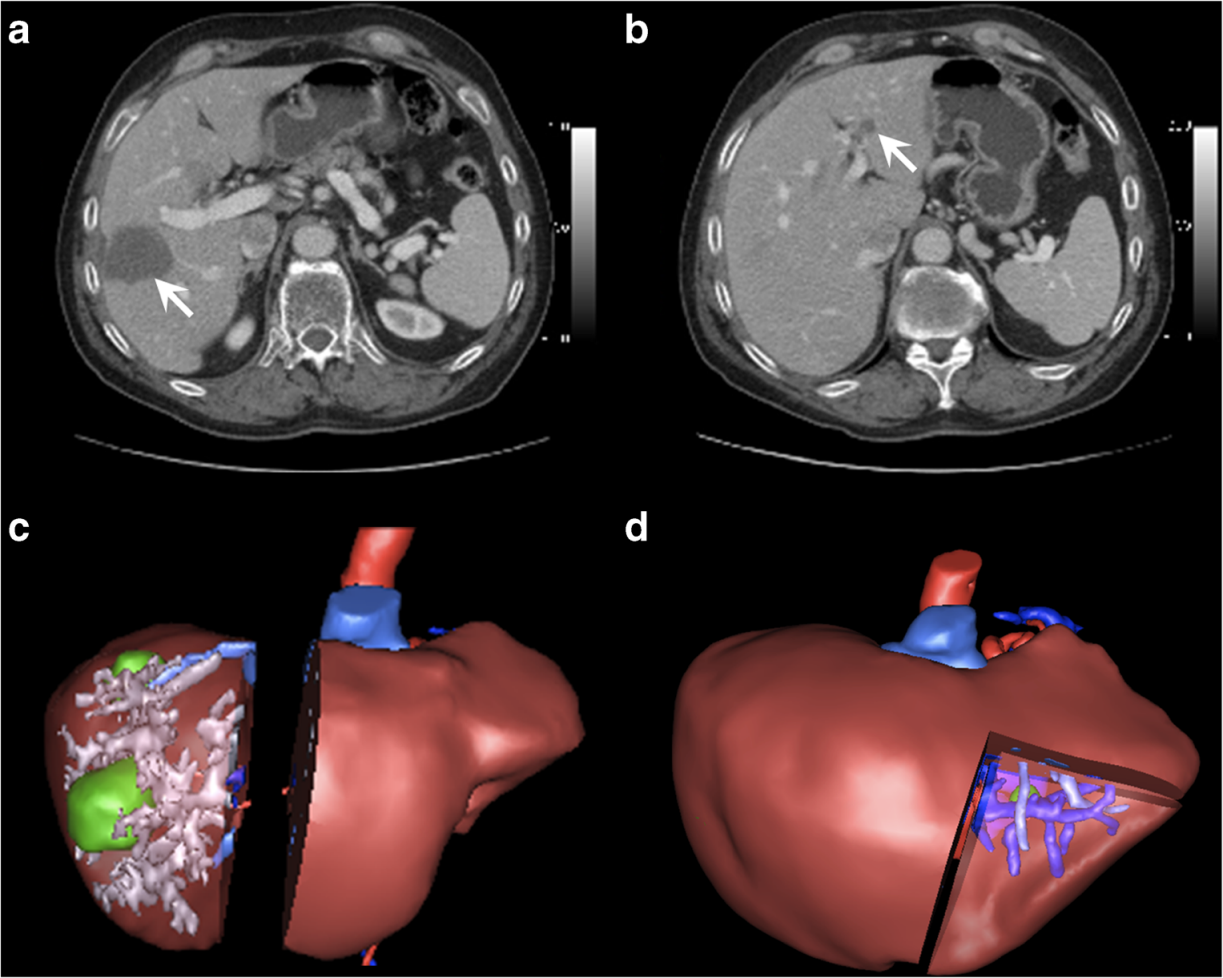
Virtual surgical planning. a Axial enhanced CT image shows a right liver metastasis centred in segment V (*arrow*). The patient also had a metastasis involving segment VII (not shown). b Axial enhanced CT image of a different patient shows a left liver metastasis in segment III (*arrow*). c Three-dimensional rendering image shows surgical planning for complete right hepatectomy including tumour and hepatic structures in patient from a. d Three-dimensional rendered image shows surgical planning for segmentectomy of segment III for patient in b. Residual hepatic liver volume after both procedures was estimated to be 27%. Right portal embolisation was thus performed before right hepatectomy. Figure courtesy of Dr. Vandenbroucke-Menu; created with 3DVSP (IRCAD, Strasbourg, France)
